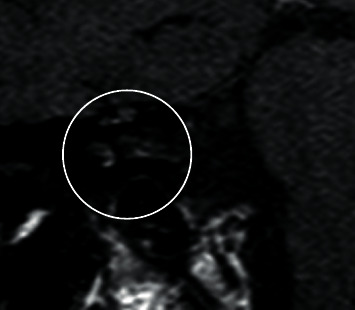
Coronal T1 MRI GAD of cerebellopontine angle before cochlear implantation of the right ear.
